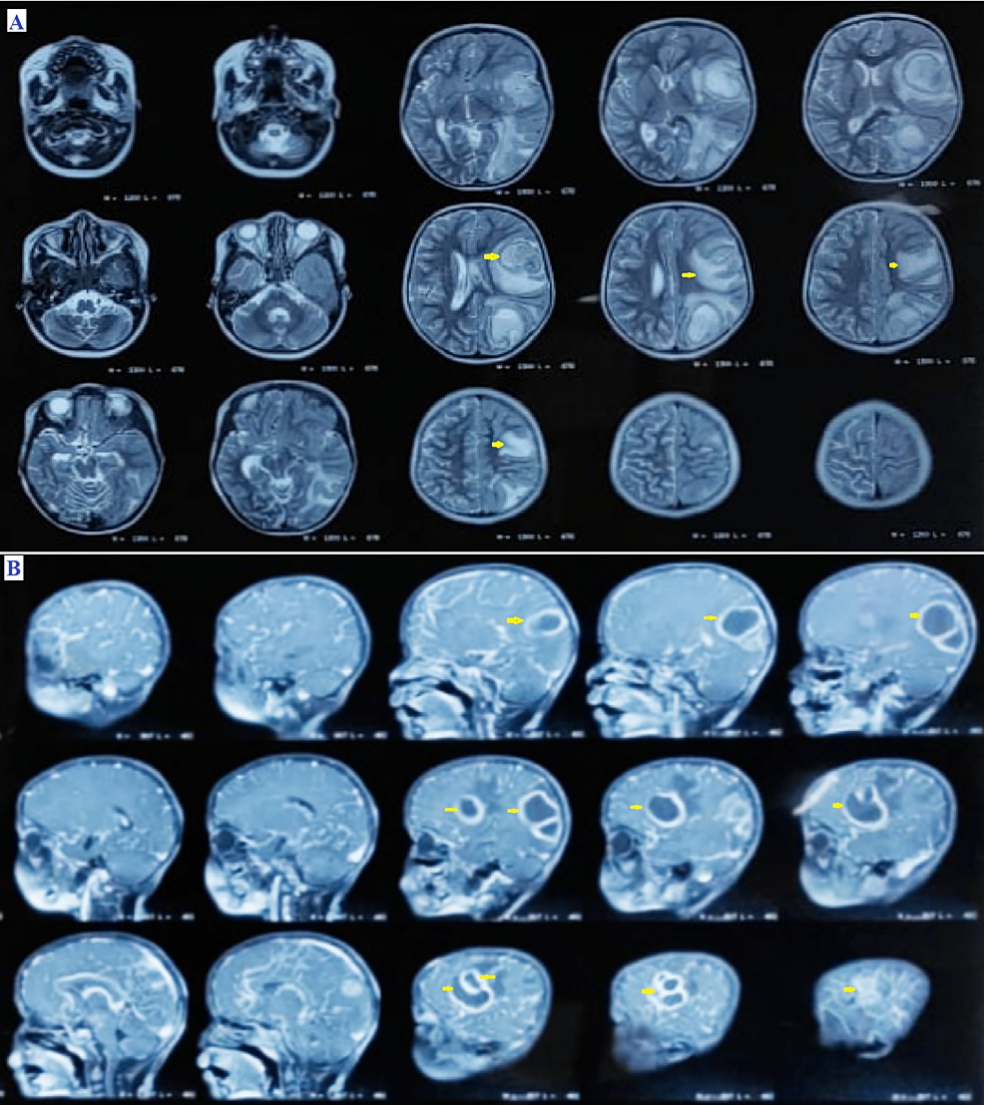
MRI (A: transverse T2 image, B: sagittal T1 image) The images show a large, well-encapsulated, thick-walled peripheral enhancing lesion (arrow) in the left parietal and frontal lobe with brilliantly restricted diffusion, moderate perifocal vasogenic edema, mass effect, and midline shift MRI: magnetic resonance imaging
